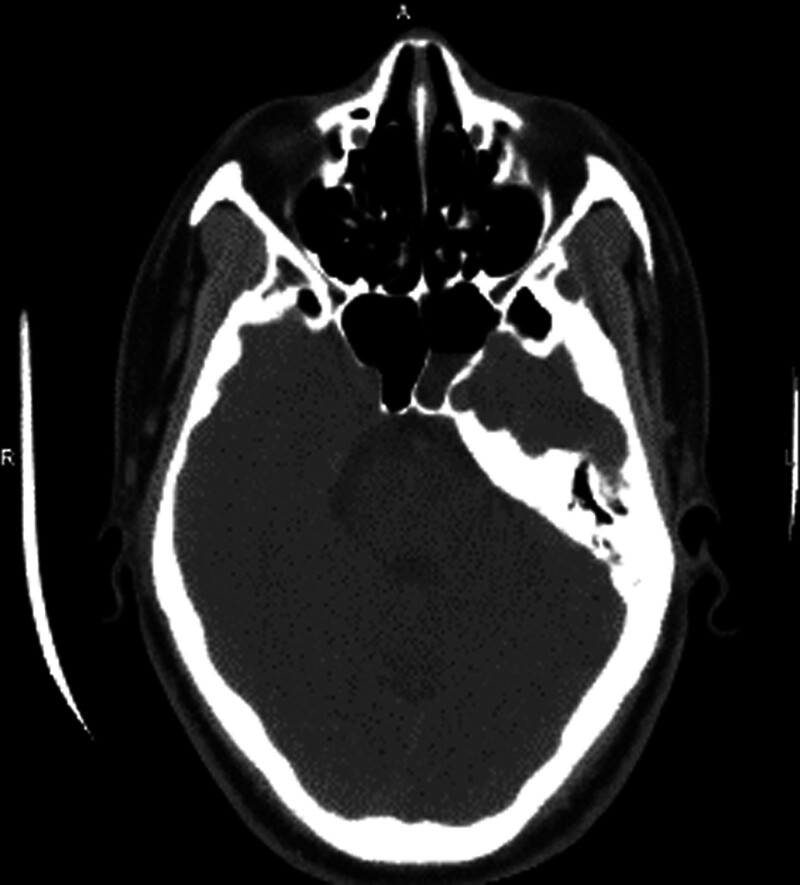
A 49-year old male patient with preoperative sinus CT horizontal position. CT = computed tomography.
